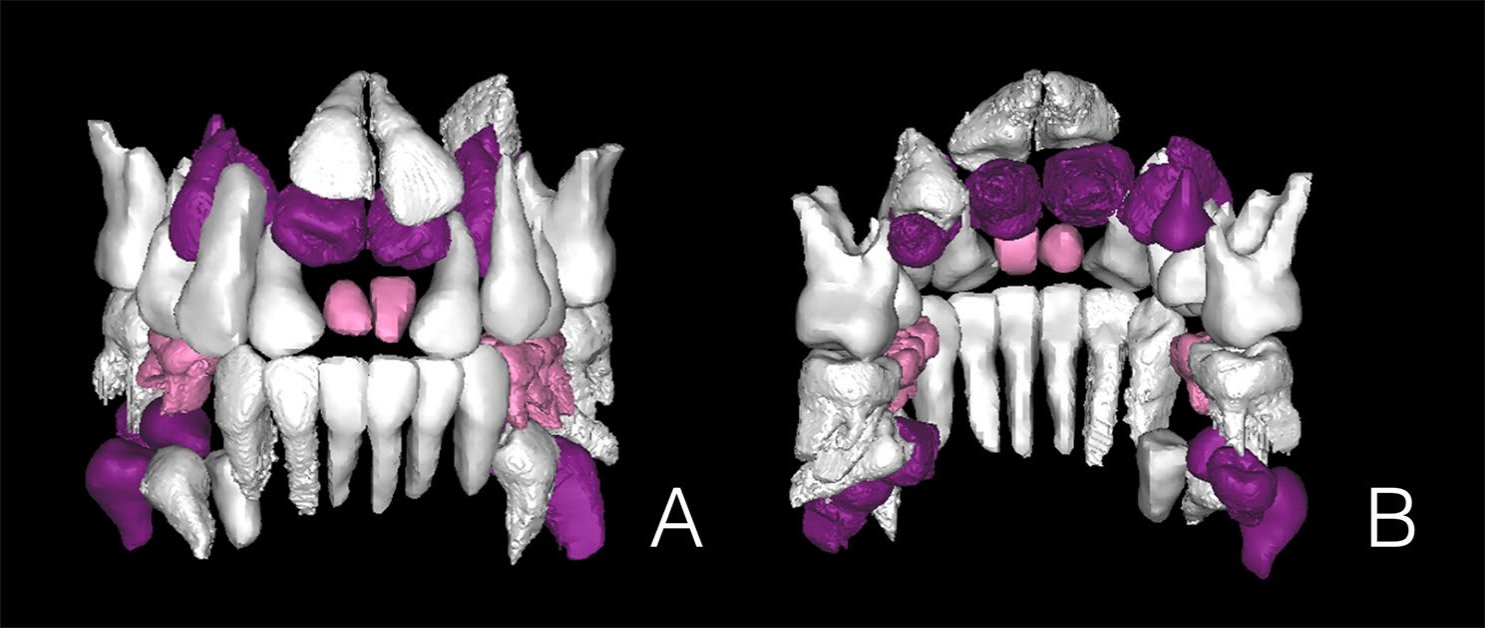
Three-dimensional images derived from cone-beam computed tomography data of teeth. (A) Labial and(B) lingual side views (Primary teeth, permanent teeth, and supernumerary teeth are shown in pink, white, and purple, respectively.)
